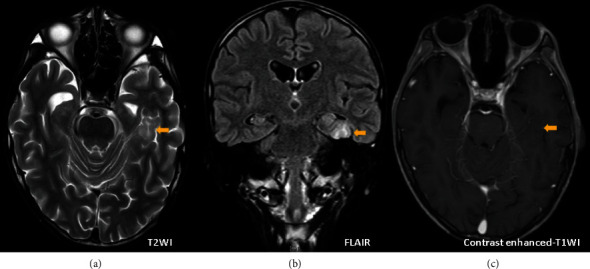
Head MRI was performed 6 weeks after admission. (a) T2-weighted image, (b) FLAIR, and (c) contrast enhanced-T1 weighted image (right) are shown. T2-weighted and FLAIR images show a localized high-signal area from the cortex to the white matter of the fusiform gyrus near the left hippocampus (arrows). It is accompanied by gray matter swelling and subcortical white matter atrophy. There was no contrast enhancement. MRI, magnetic resonance imaging; FLAIR, fluid attenuated inversion recovery.
